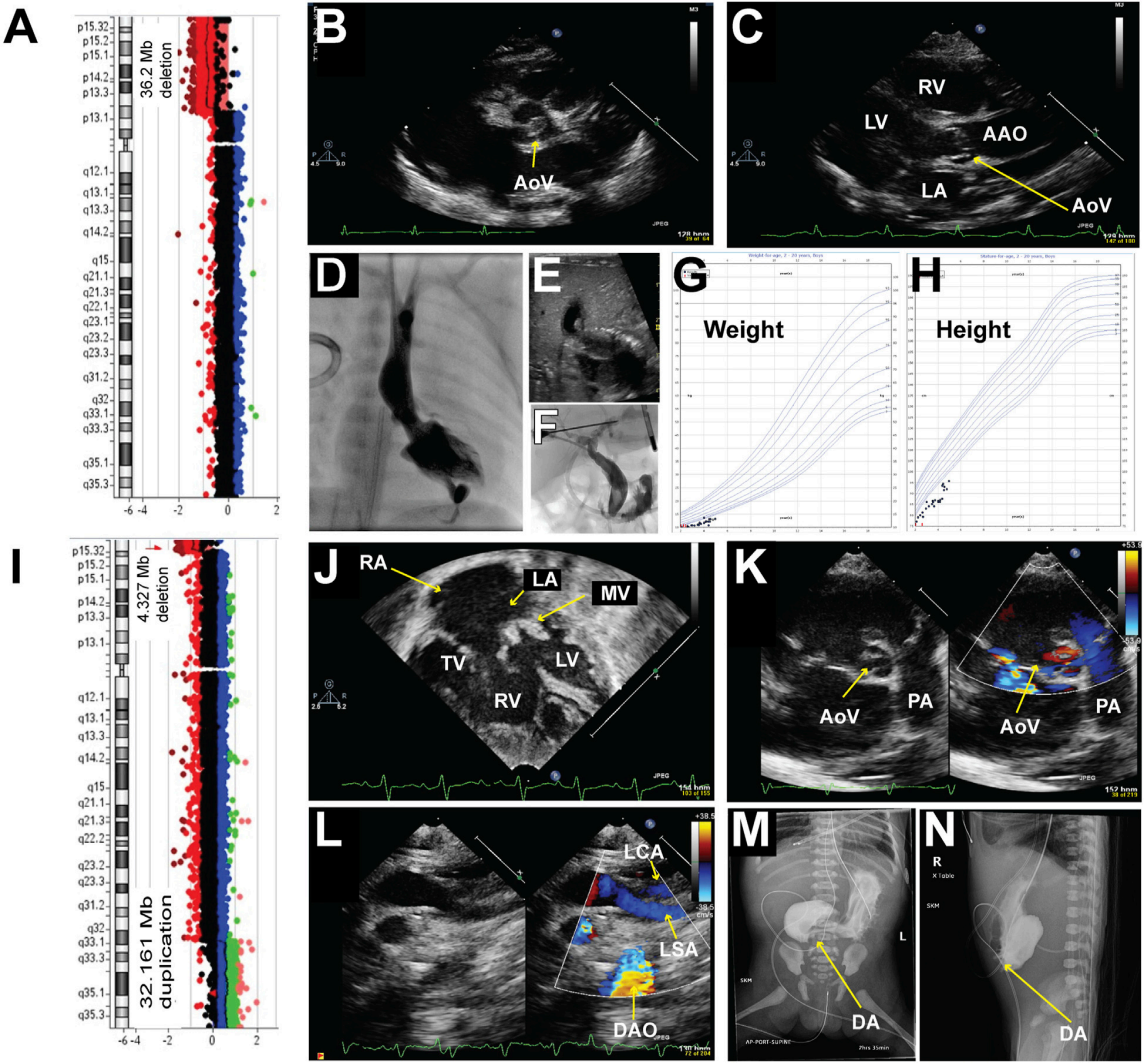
Chromosomal abnormalities and imaging from patients 1 and 2. Patient #1 (A–H) A Chromosomal microarray detected a 35.2 Mb deletion at 5p, specifically arr [GRCh37] 5p15.33p13.2 (22149_36232545) x1. (B–C): Representative echocardiogram imaging demonstrating the patient’s dysplastic aortic valve and post-stenotic dilatation of the ascending aorta. (D) Left ventriculogram demonstrating an apex-forming left ventricle with a hypoplastic aortic valve. The aortic valve annulus measured 4.46 mm in the anterior-posterior projection with mild post-stenotic dilation of the ascending aorta. A posterior pseudoaneurysm was noted with a small track extending apically through the myocardium. (E) Representative abdominal ultrasound image demonstrated dilation of the left intrahepatic biliary system as well as the intrahepatic common bile duct with gall sludge within the intrahepatic common bile duct. (F) An injection of contrast from a micro-puncture needle into a left hepatic duct filled a mildly dilated segmental biliary branch and communication with the dilated common hepatic and biliary ducts. (G) Growth chart of weight. (H) Growth chart of height. Patient #2 (I–N) (I) Chromosomal microarray detected a 4.327 Mb deletion at 5p, specifically arr [GRCh37] 5p15.33 (22149_4349495) x1, and a 32.161 Mb duplication at 5q, specifically arr [GRCh37] 5q32q35.3 (148535314_180696806) x3. (J–L): Representative echocardiogram imaging demonstrating a hypoplastic left ventricle, dysplastic and thickened mitral valve, large secundum atrial septal defect (ASD), large ventricular septal defect (VSD), and a bicuspid aortic valve with thickened leaflets. Color flow Doppler in image (L) showed a lack of continuity between the left subclavian artery and the descending aorta consistent with interrupted aortic arch type (A). (M–N): Upper GI with small bowel follow-through demonstrating opacification of the stomach and blind-ending proximal duodenum is consistent with duodenal atresia. AAO = Ascending Aorta, DAO = Descending Aorta, LA = Left Atrium, LV = Left Ventricle, LSA = Left Subclavian Artery, MV = Mitral Valve, PA = Pulmonary Artery, RA = Right Atrium, RV = Right Ventricle, TV = Tricuspid Valve.
